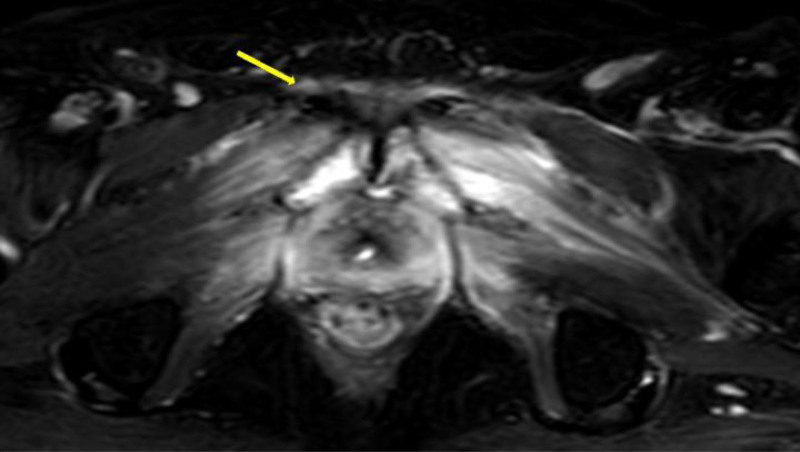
Contrast-enhanced pelvic magnetic resonance imaging. The yellow arrow indicates the infected area.
